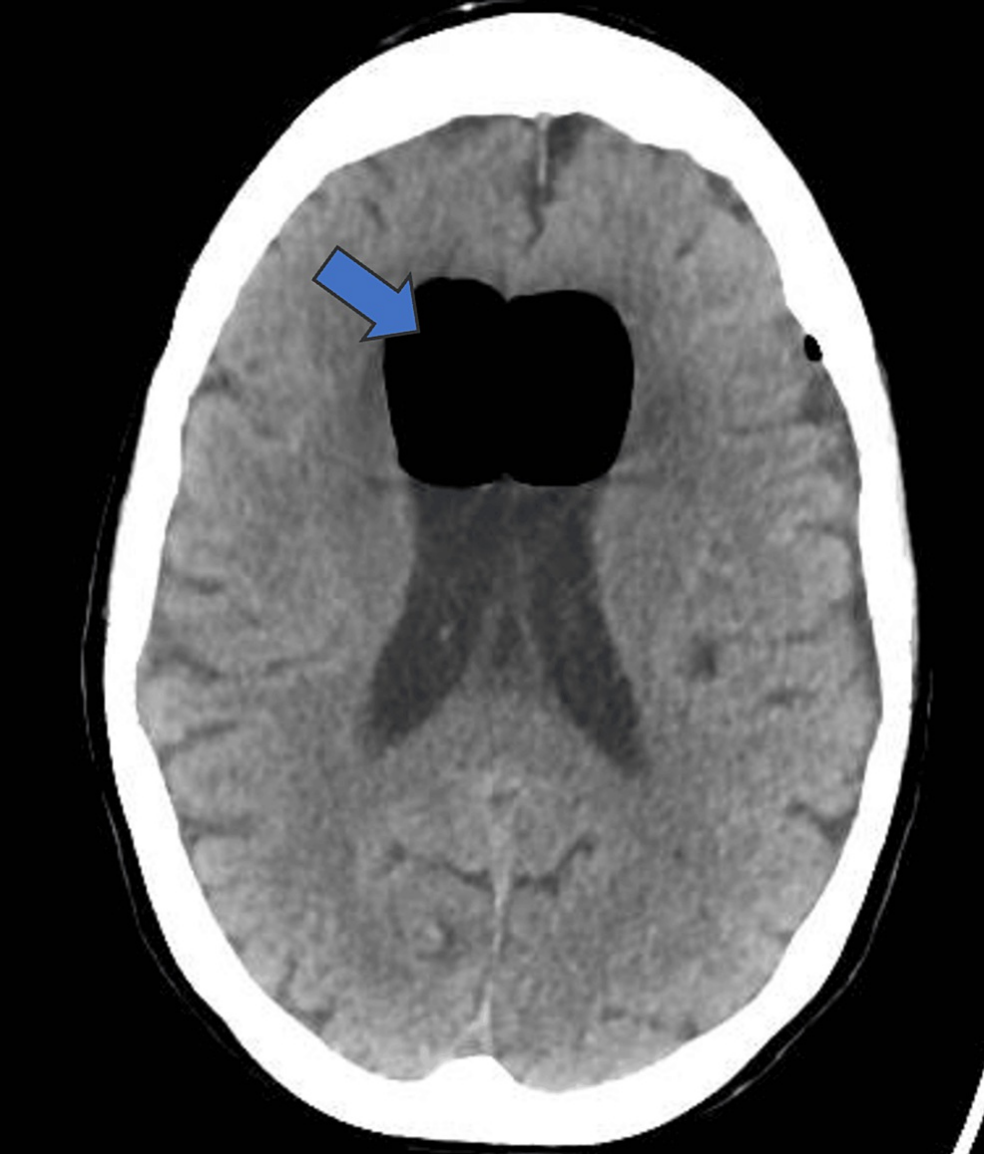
CT scan head showing pneumocephalus in the intraventricular system (arrow)
